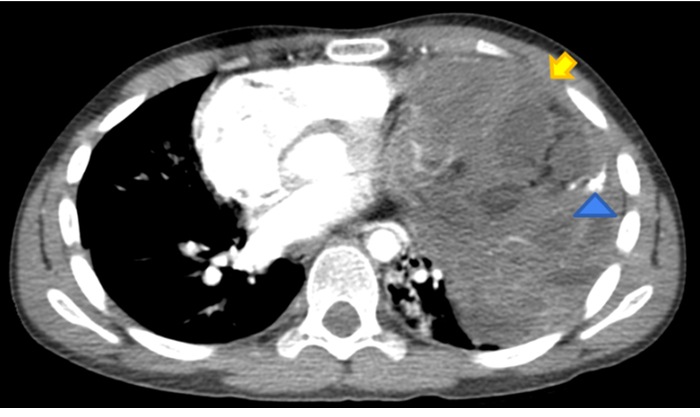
Axial contrast-enhanced chest CT performed at diagnosis showing a large (11×9×15 cm) left-side well-demarcated inhomogeneous soft-tissue density mass (*yellow arrow*) with calcification (*blue arrow*) displacing the adjacent structures. Radiologic features were consistent with teratoma.
